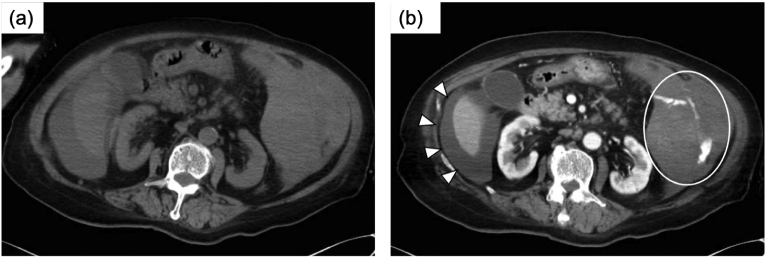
CT of the abdomen on the 28th hospital day Simple CT (a) and contrasted CT (b) of the abdomen showed hematogenous ascites effusion (arrowhead) and extravasation from the caudal side of the spleen implicating active bleeding from the inferior branch of the splenic artery (white circle).
